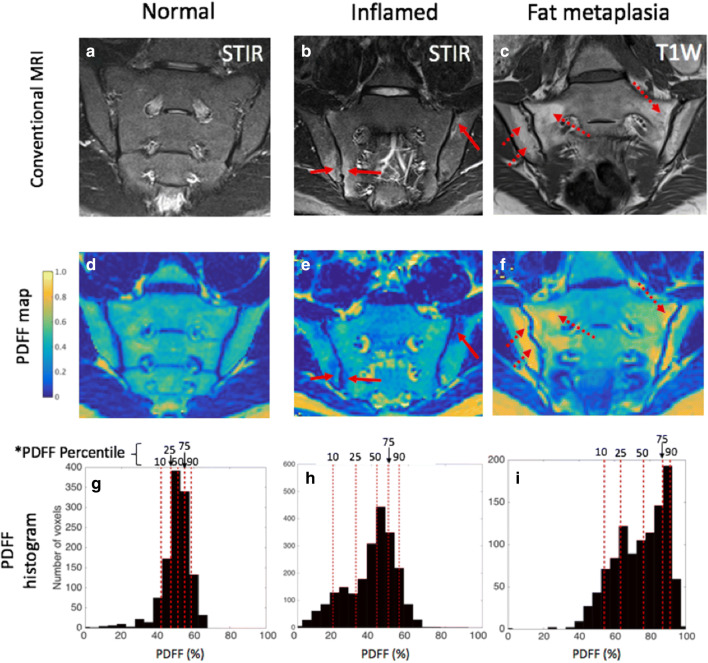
Examples of histograms generated using the BEACH tool. Conventional MR images (a–c), PDFF maps (d–f) and PDFF histograms (g–i) are shown. In the normal patient’s histogram (g), PDFF values are clustered around 50%, corresponding to normal marrow. In the patient with inflammation, a number of low-PDFF pixels have emerged in the histogram (h). In the patient with fat metaplasia, there is an upward shift in PDFF values, with a large number of high-PDFF pixels (i)
